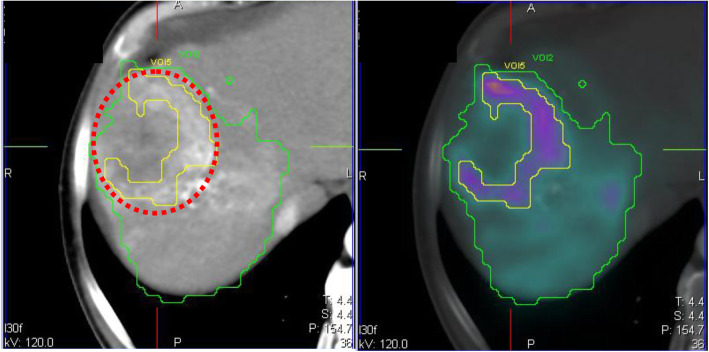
Segmentation of a lesion with non-perfused core, based on ^99m^Tc-MAA SPECT image (in colour on the right) co-registered to a contrast-enhanced CT (in black and white in both panels) (manual rigid co-registration). The lesion is segmented both on CT (red dotted line) and nuclear medicine (yellow line) images. The region “liver perfused” with radioactive particles is segmented on nuclear medicine images (green line). For toxicity prediction, the CT-based segmentation (red dotted) should be considered as lesion has to be excluded from the non-tumoural whole liver volume
